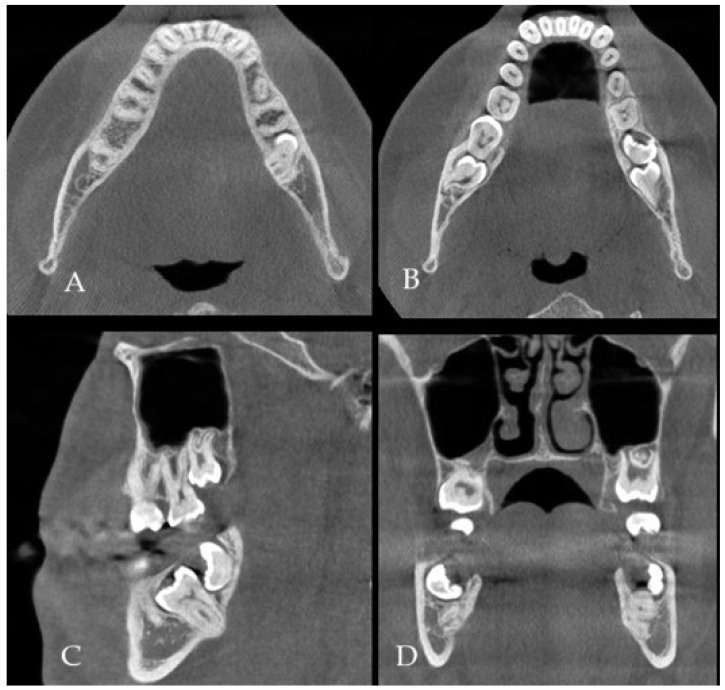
(A) CBCT (cone beam computed tomography scans: CBCT 20 × 20 cm FOV (field of view) imaging protocols based on RayScan S 5471.3 mGy (Ray Company Co., Ltd., Samsung 1-ro, Hwaseong-si, Gyeonggi-do, Republic of Korea), CBCT evaluation was carried out in RAYSCAN S without slicing, with an average thickness between 0.070 mm and 0.3 mm) is a very valuable diagnostic tool to estimate bone status, shape, and its anatomy, as well as the boundaries of the dentigerous cyst (DC) and impacted or retained teeth. The authors used a suitable software for CBCT evaluation (RadiAnt Dicom Viewer Software version 2020.2.1 Medixant, Poznań, Poland). In some cases, after the removal of any teeth and cysts or tumors within the jaw bones, it is quite common that due to the bone loss, a fracture of the mandible or some degree of bone mobility might be found. Some authors in those cases suggest not only a prophylactic bone plating with titanium miniplates but also applying some additional bone grafts to improve bone volume and avoid any early or late bone fracture. Both a bone fracture and its mobility after the removal of a certain portion of bone might not only cause its delayed healing, resulting in a complete or displaced fracture but also promote some granulation tissue, local inflammation, or fistula occurrence. A good CBCT evaluation is helpful in the planning of the scope of each surgery and in predicting the occurrence of some worrisome aspects, especially related to bone loss, fracture occurrence, and planning for any orthodontic treatment. The type of bone used (xenograft, allograft, autograft) could be used in various bone defects. In the presented case, because the occurrence of totally impacted teeth 37 and 38 with a presence of DC, a decision was made to use an allograft bone ((A–D), where (A,B) axial scans, (C)—sagittal view and (D)—coronal view in CBCT). Because of the level of teeth 37 retention, its proximity to the lower mandible border, and perforation of both lingual and buccal plates, a larger amount of bone was necessary to stabilize the mandible left basis deficit and improve its postoperative height and width. On the other hand, a so-called PMP (prophylactic/preventive mandible plating) during the removal of more locally advanced jaw lesions is a good solution. A panoramic radiograph (panx) or a classic CT does not show the close boundaries between tooth roots, inferior alveolar nerve (IAN), cortical plate, and the scope of DC occurrence, but the panx role for screening is quite good [1,2,3].
